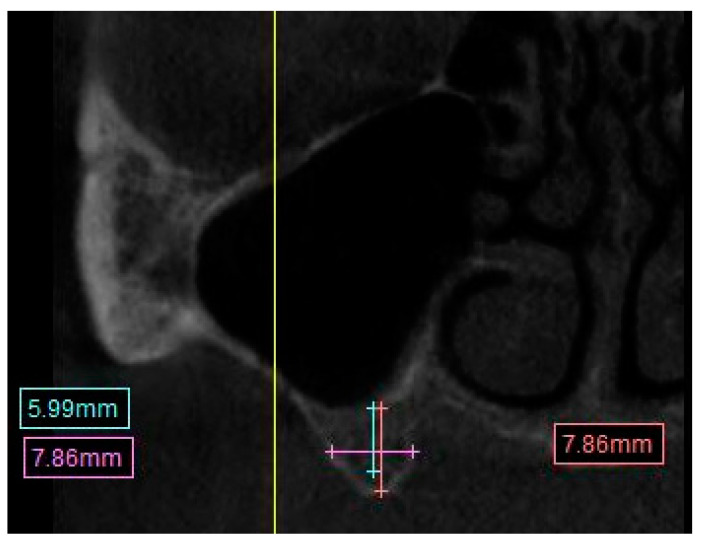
CBCT image showing measurement calculation for the distance from maxillary ridge crest to the floor of the sinus.
